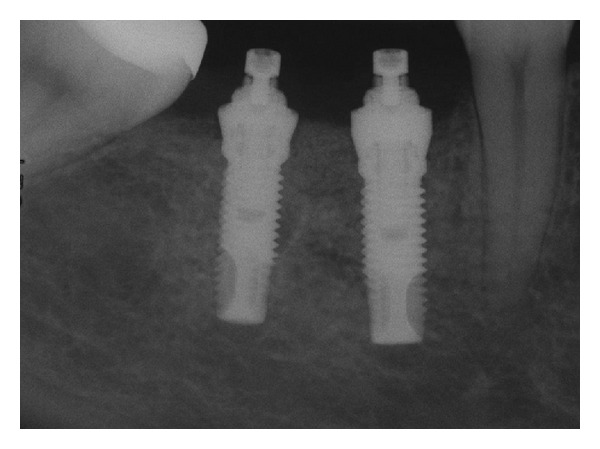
Periapical radiograph at abutment connection.
